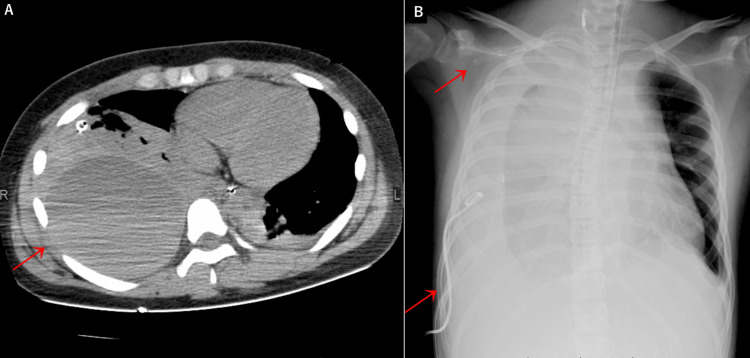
Representative images of a right hemothorax and a large right extrapleural hematoma (A) Chest CT scan showing a right hemothorax and a large right extrapleural hematoma (red arrow). (B) Chest X-ray showing additional stenting of the right subclavian artery and drainage of the hemothorax and extrapleural hematoma (red arrow).
